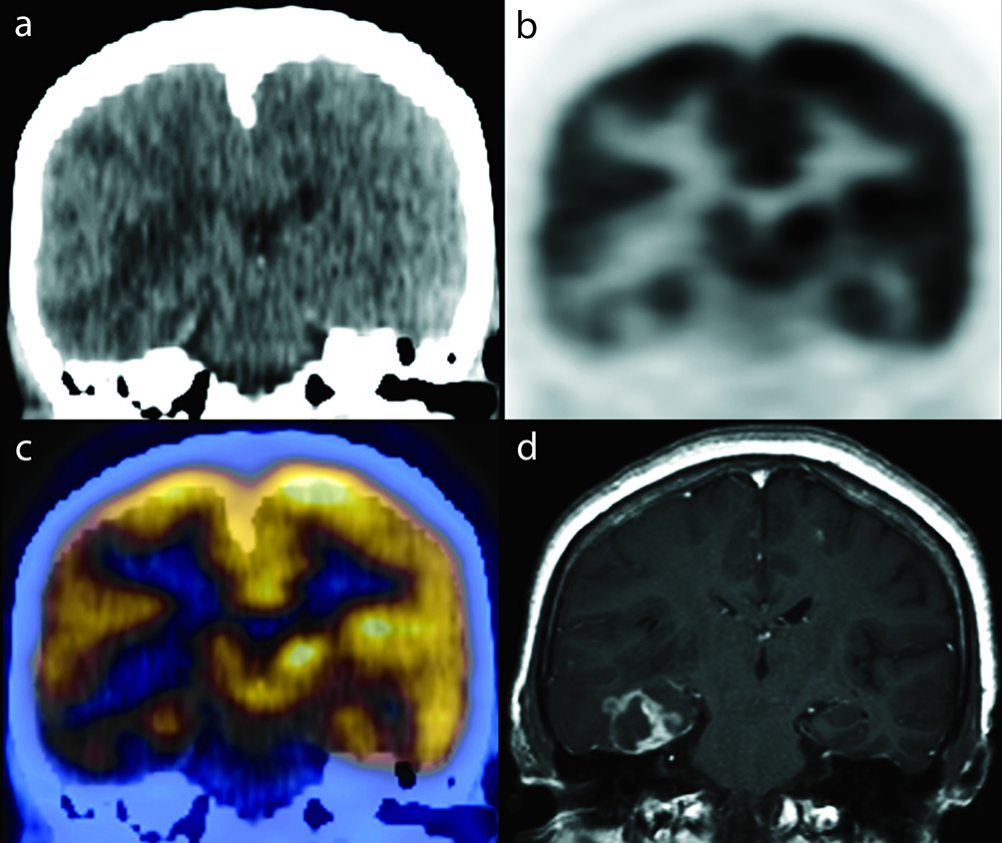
(a) Coronal CT, (b) positron emission tomography, (c) positron emission tomography/CT fusion and (d) MRI *T*_1_ weighted post-gadolinium images. The largest single lesion in the right temporal lobe measured 2.4 × 1.9 cm in the coronal plane.
